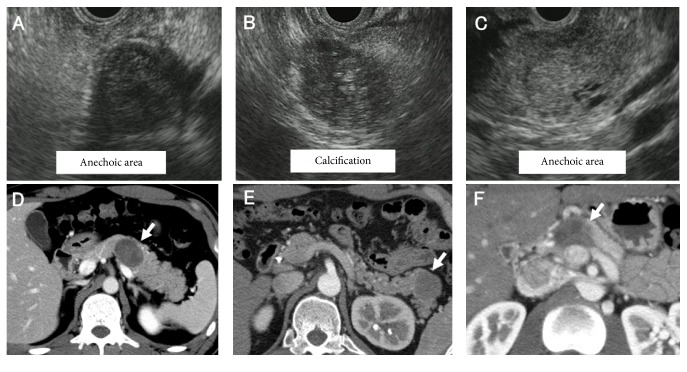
Endoscopic ultrasound and computed tomography findings of three young male patients with solid pseudopapillary neoplasms. The endoscopic ultrasound and computed tomography findings of three young male patients with solid pseudopapillary neoplasms are shown. Solid and hypoechoic masses with anechoic areas were observed in (A) Case  1 and (C) Case  3. Internal calcification was observed in (B) Case  2 by endoscopic ultrasound. Computed tomography showed low-density tumors with slight enhancement at the margin in all three cases.
